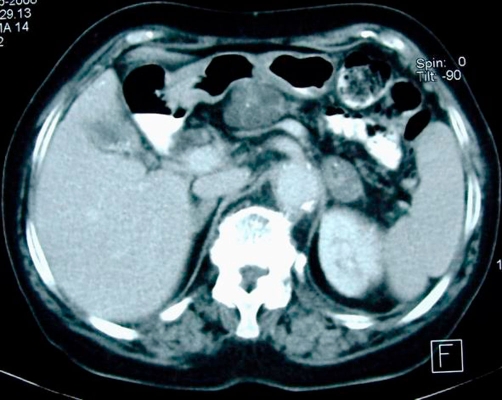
Contrast enhanced axial CT shows a well defined, lobulated cystic mass with internal septations at the neck of pancreas. Concomitant findings are cholelithiasis and an adenoma of the left adrenal.
